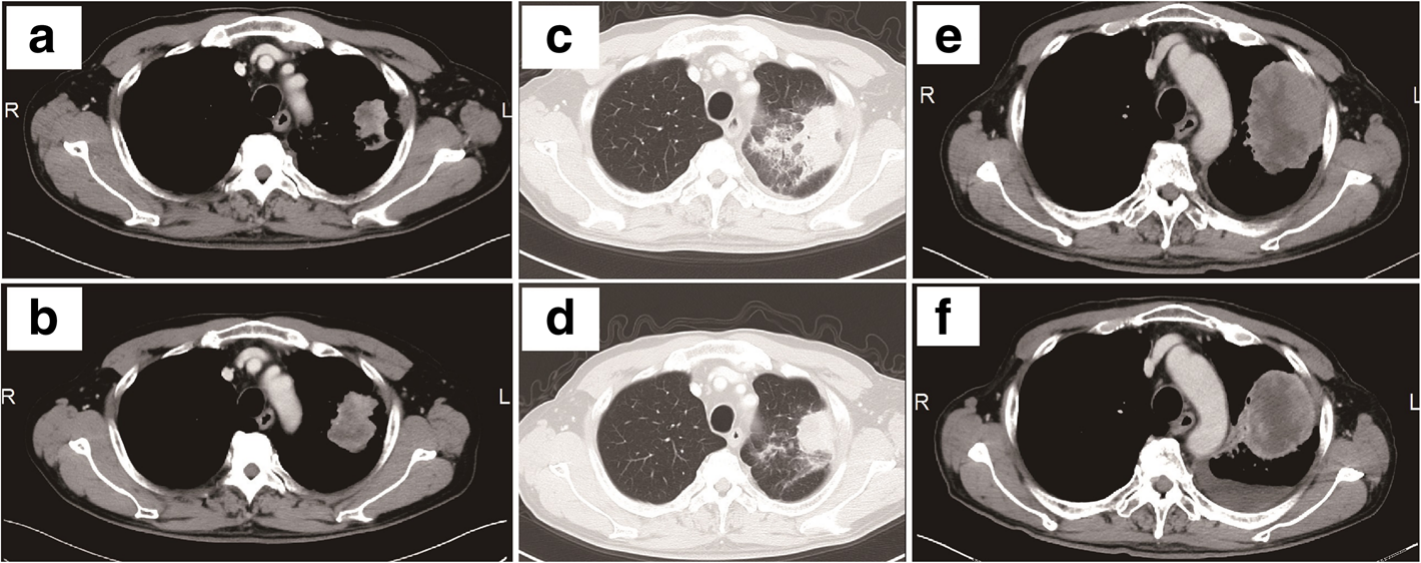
Radiographic results before and after ICP inhibitors. The CT scan shows the primary lesion in the right upper lobe before the first-line ICP inhibitor (a), SD after 2 months accompanied by alveolitis (c, d), and enlarged after 4 months (b). After the failure of five lines of chemotherapy (e), the primary lesion responded to 3 cycles of nivolumab (f)
